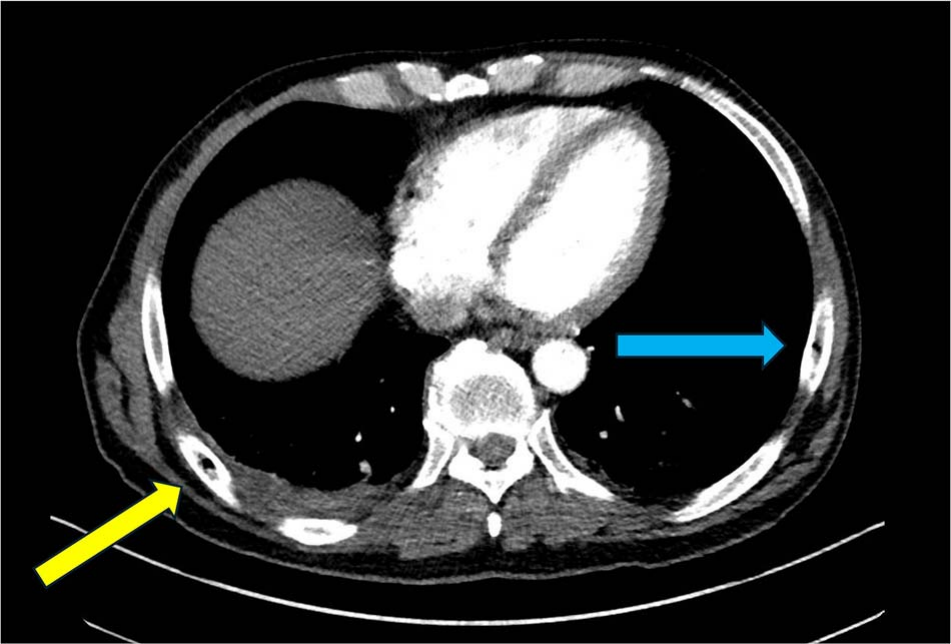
CT chest—axial plane. Intraosseous gas visualized in the right sixth rib (yellow arrow, left) and left seventh rib (blue arrow, right).
